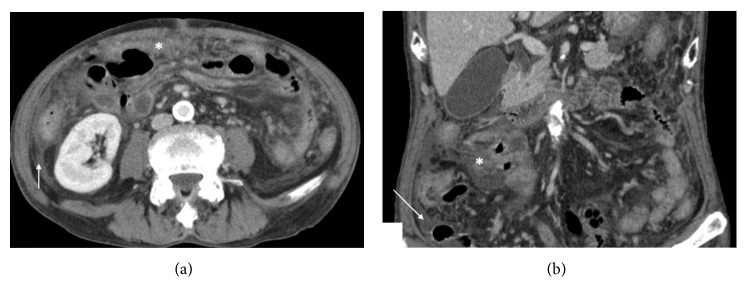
Abdominal computed tomography (CT) scan taken again after the patient showed the peritoneal irritation sign, revealing an increased CT value of the greater omentum (^∗^) and ascites (→). However, no infectious focus or neoplasms are observed.
